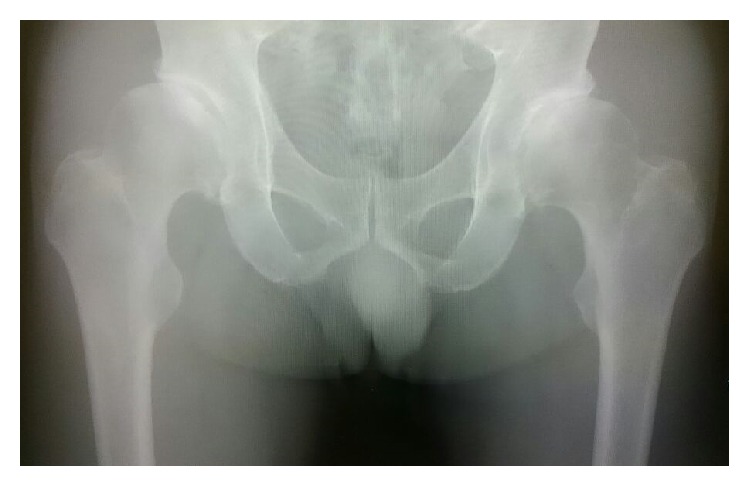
Preoperative anteroposterior X-ray showing bilateral osteoarthritis of the hip.
